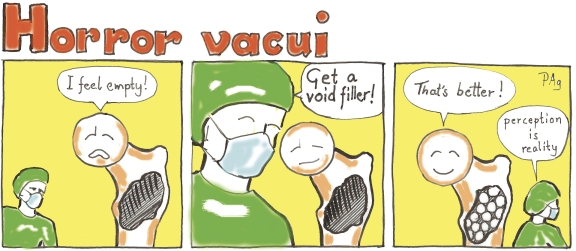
It is likely that the radiographic appearance of voids in the bone steer the thoughts of action-inclined observers, who think that an abnormality in itself is a call for correction. This is really fear of emptiness (*horror vacui*), transformed into a surgical indication. (Actually, *horror vacui* is not a psychological term, but belongs to Aristotelean physics). The same psychology probably lies behind the idea that bone replacement materials should be resorbable: when the void is filled with a material, radiographs still look abnormal. We forget that patients don't care about how their radiographs appear—but about their interpretation, for which we are responsible. Let's hope that the papers by Hirn et al. and Yanagawa et al. get all the attention they deserve. As Göran Bauer, previous Editor in Chief of Acta Orthopaedica, often said: “Retreat from overtreatment”!
